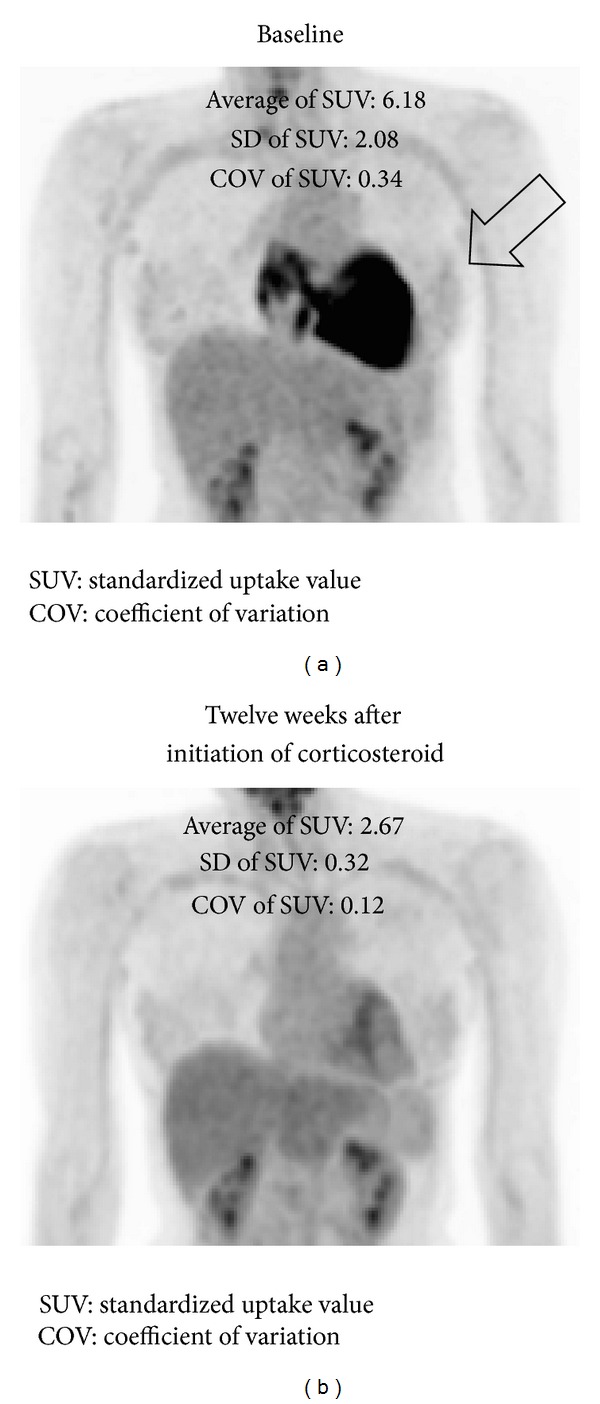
^18^F-FDG PET before and after corticosteroid therapy. (a) ^18^F-FDG PET image (3D maximum intensity projection) before therapy. (b) After administration of 30 mg/day prednisone for 12 weeks, FDG uptake (arrow) has almost disappeared. ^18^F-FDG PET: ^18^F-fluoro-2-deoxyglucose positron emission tomography. SUV: standardized uptake value, COV: coefficient of variation.
